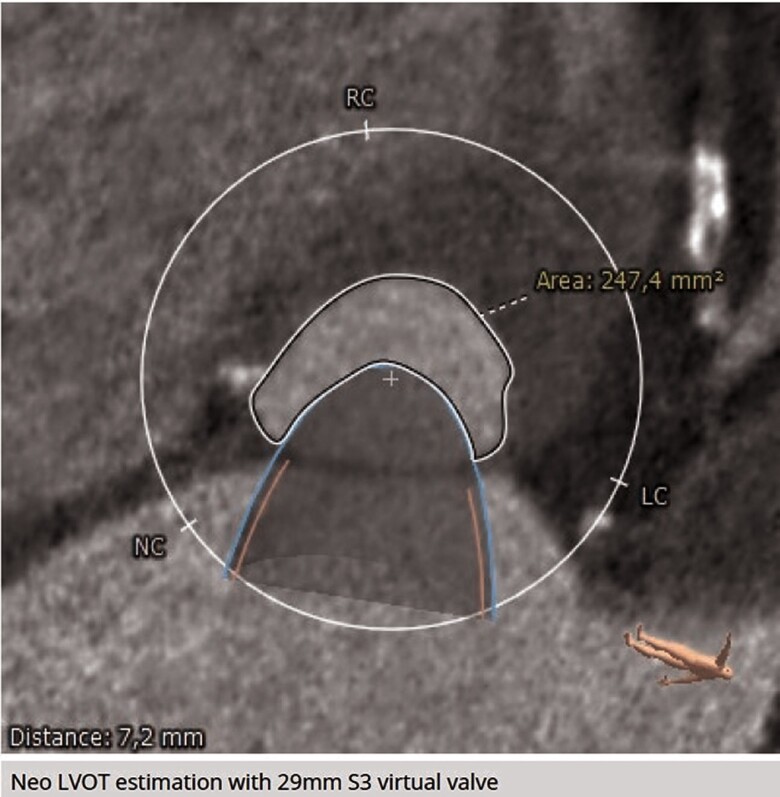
Virtual estimation of neo-left ventricular outflow tract area for the Edwards SAPIEN 3 valve on multiplanar cardiac-gated computed tomography.
